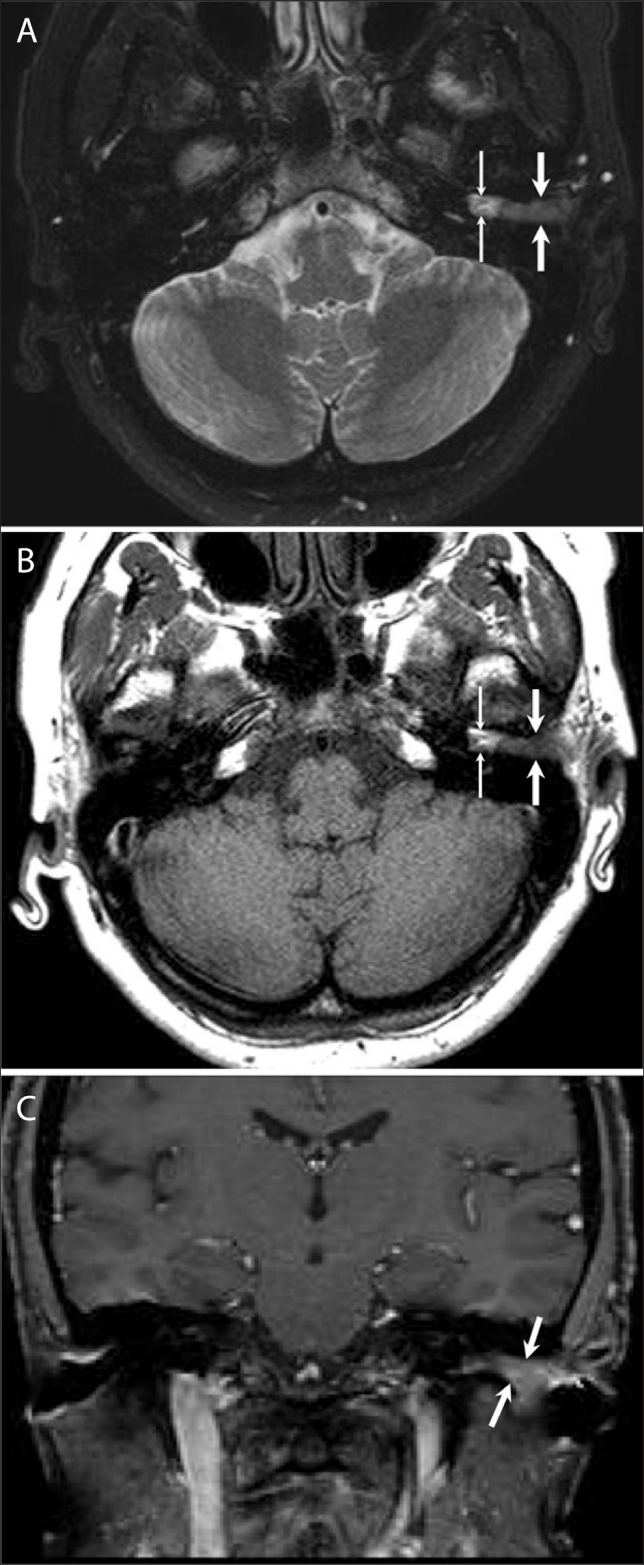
Axial fat-suppressed T2-weighted (A) and T1-weighted (B) images show left EAC soft tissue mass (thick arrows) that is iso-intense with muscle on T1-weighted image, slightly hyperintense on T2-weighted image. There is a small, T1- and T2 hyperintense, hemorrhagic fluid collection (thin arrows) between the mass and the tympanic membrane. Post-contrast coronal fat-suppressed T1-weighted (C) images show intense contrast enhancement in the mass.
